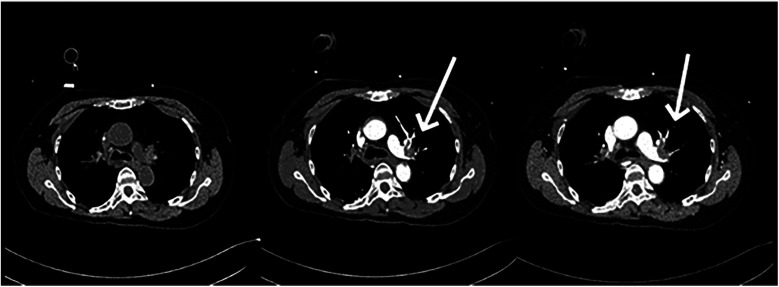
Non-contrast CTA of the pulmonary artery, arterial phase, venous phase.
